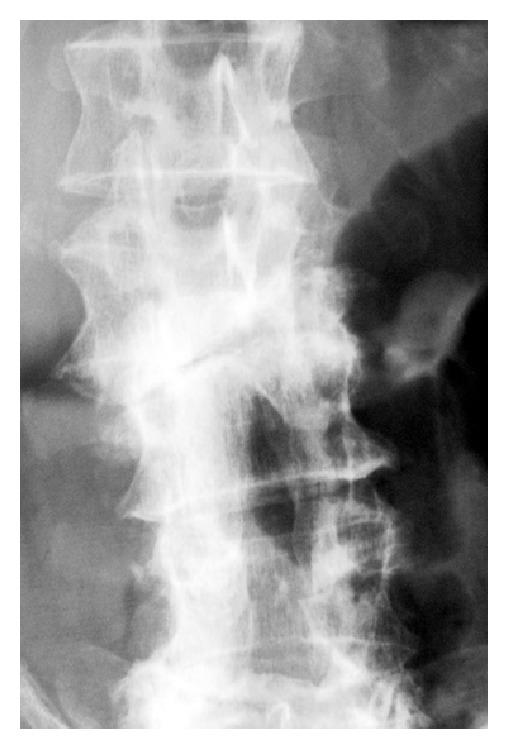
Anteroposterior radiographs showing marked narrowing of L2-L3 disc space with some endplate destruction associated with new bone formation and a severe lateral dislocation of the L2/L3 joint (Case 1).
